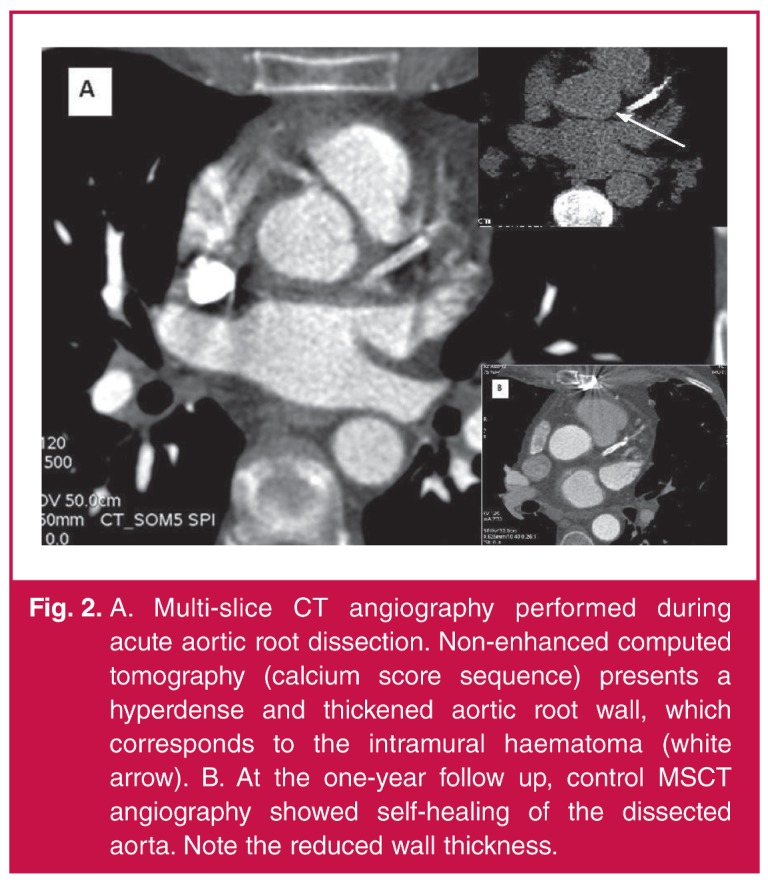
A. Multi-slice CT angiography performed during acute aortic root dissection. Non-enhanced computed tomography (calcium score sequence) presents a hyperdense and thickened aortic root wall, which corresponds to the intramural haematoma (white arrow). B. At the one-year follow up, control MSCT angiography showed self-healing of the dissected aorta. Note the reduced wall thickness.
